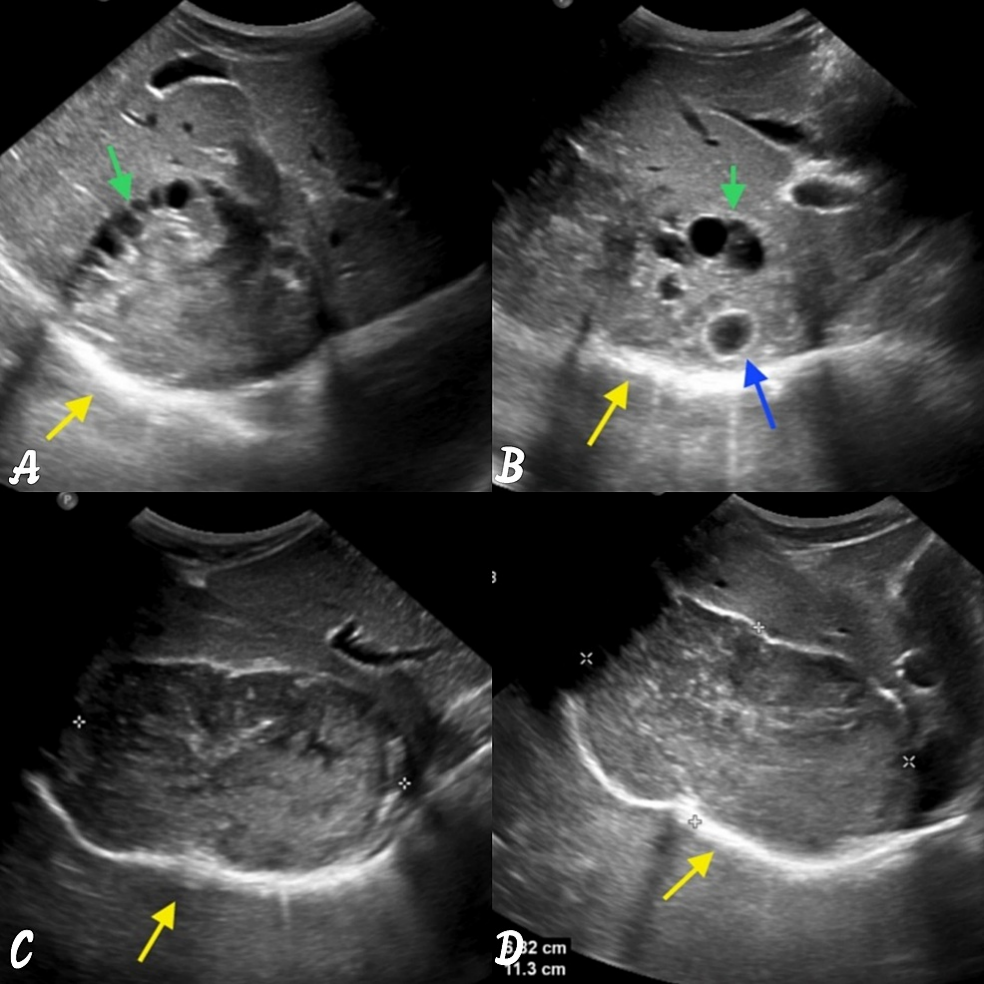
Ultrasonographic images showing multiple daughter cysts. (A) The cysts are located along the periphery giving spoke wheel appearance (green arrow). (B) Few of the daughter cysts show echogenic walls likely due to calcification (blue arrow). (C and D) The main cyst shows heterogeneous echotexture and is abutting the right hemidiaphragm (yellow arrow) and lies in segments VII and VIII.
